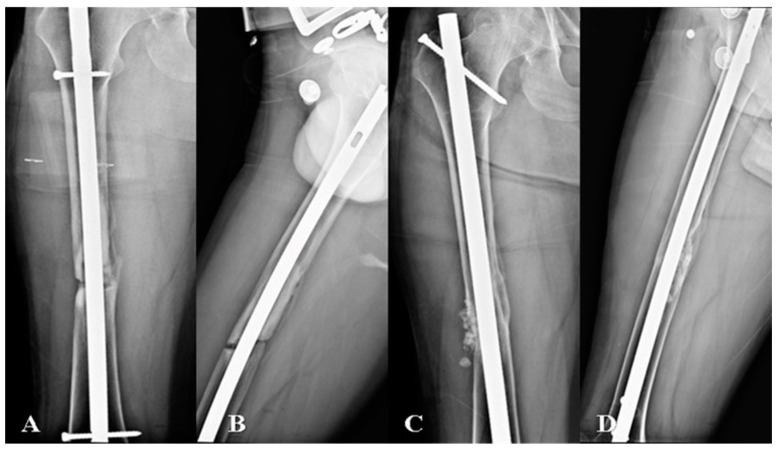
Radiological images showing a 34-year-old patient with atrophic nonunion of the femoral shaft who underwent exchange nailing. The images included preoperative radiographs: (A) anteroposterior radiograph and (B) lateral radiograph, as well as postoperative follow-up; (C) anteroposterior and (D) lateral radiographs.
